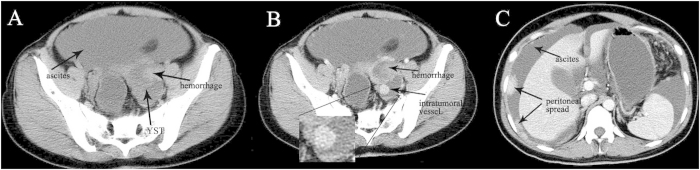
27-year-old young woman with ovarian yolk sac tumor. A Precontrast CT scan shows a high density lesion within a solid-cystic mass (black arrow). Massive ascites is seen (black arrow). B This high density lesion has no enhancement on post-contrast CT image, which indicates the lesion is hemorrhage (black arrow). Heterogeneous marked enhancement of the tumor and enlarged intratumoral vessels are detected (black arrow). C Multiple peritoneal metastases (black arrows) with marked enhancement and massive ascites (black arrow) are seen.
